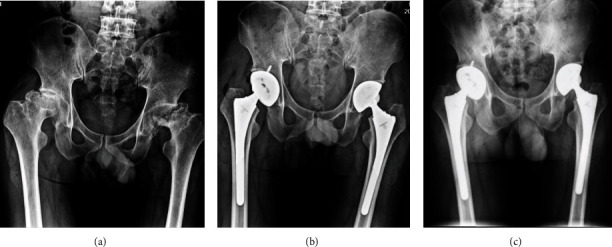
(a) Preoperative X-ray of a 69-year-old male patient with bilateral femoral head necrosis ARCO IV (HIV patients) who underwent bilateral SuperPATH approach. (b) The results of X-ray 1 week after surgery showed that the reconstruction of the length and eccentricity of both lower limbs was good. (c) X-ray at 6 months after surgery.
